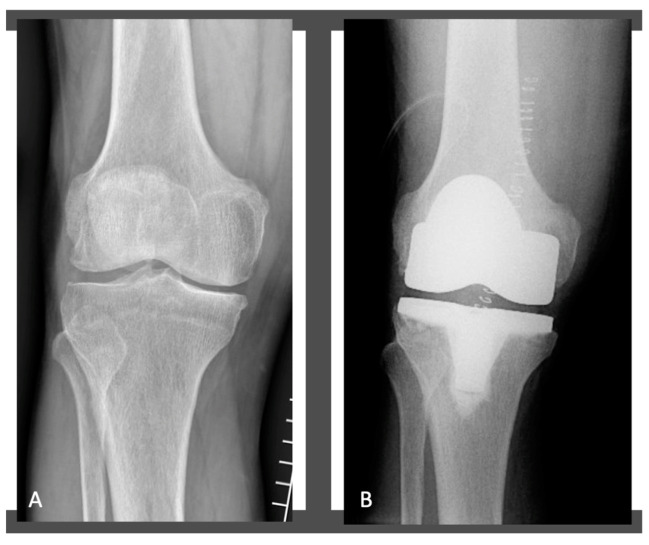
Radiographs of the patient’s knee before (A) and after KA MP-TKA (B).
